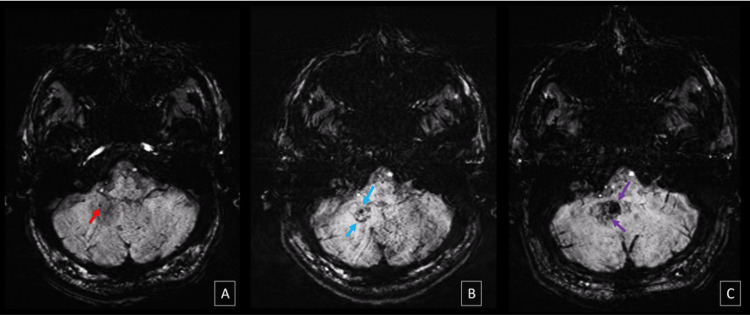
Axial SWI of a 63-year-old male patient with brain metastasis from a neuroendocrine tumor of the esophagogastric junction SWI: Susceptibility-weighted imaging; MRI: magnetic resonance imaging; SRS: stereotactic radiosurgery In the baseline MRI (A), prior to SRS, a solid lesion with punctate central magnetic susceptibility areas is observed (red arrow). In MRI-2 (B) at three months, there is a reduction in lesion size with an increase in magnetic susceptibility areas, showing central and peripheral distribution (blue arrows). In MRI-3 (C) at six months, a greater central and peripheral component of magnetic susceptibility areas is observed (purple arrows)
